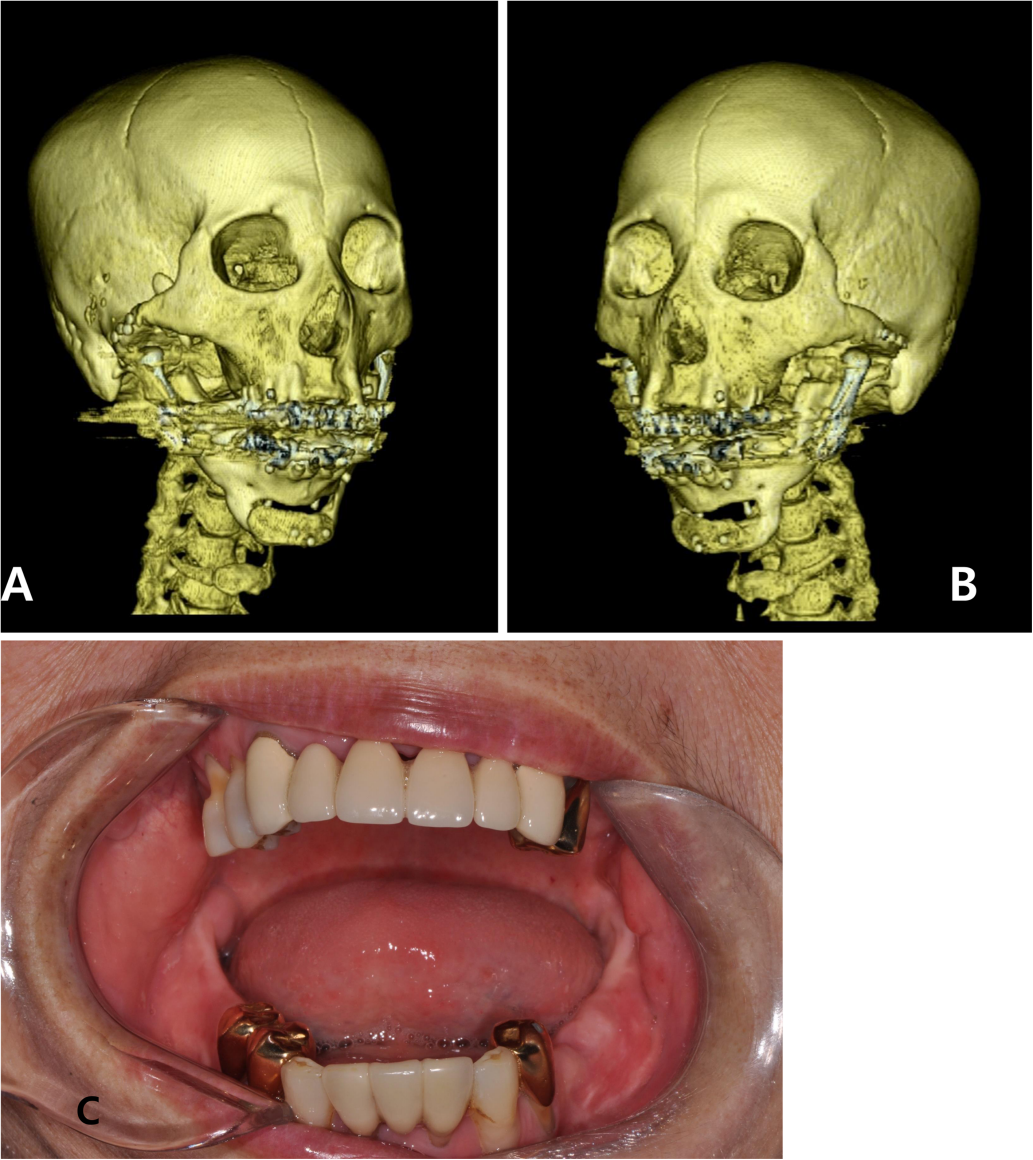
The 3D-CT images taken 2 months after surgery (a, b). Clinical photos of 2 months after surgery (c). The amount of maximum opening increases up to 30 mm
